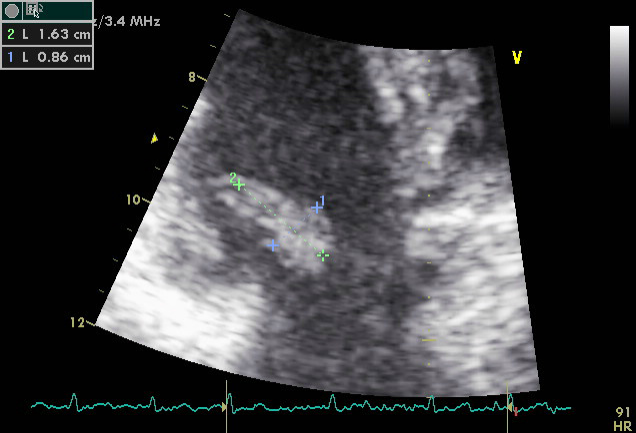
Echocardiographic image of tricuspid mass.
